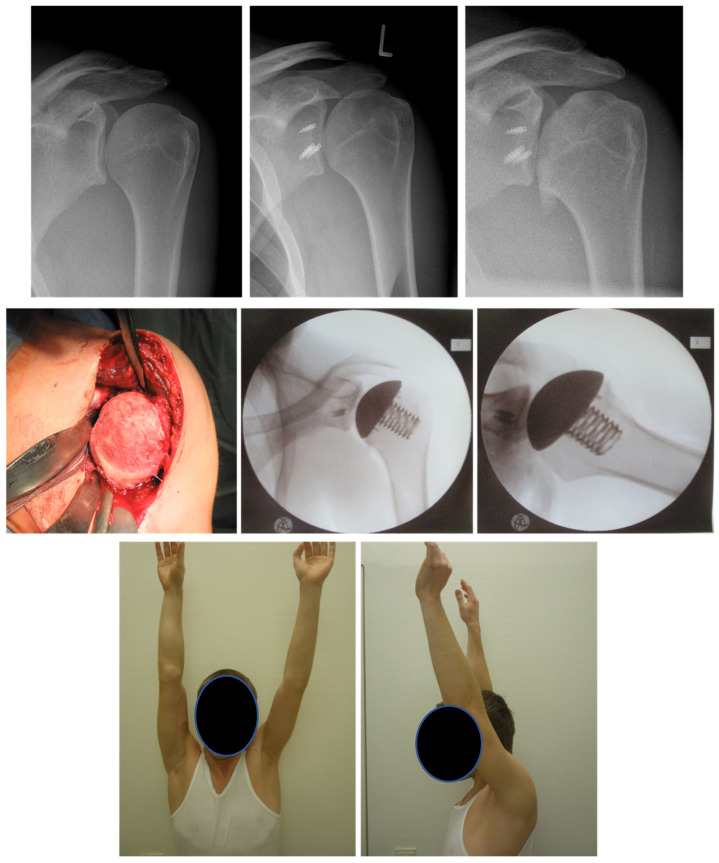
22-year-old patient with dislocation arthropathy after instability surgery treated with stemless prosthesis. First row: radiographs showing OA after shoulder instability surgery. Second row: intraoperative pictures of hemiprosthesis. Third row: Physical examination and shoulder function at final follow-up.
